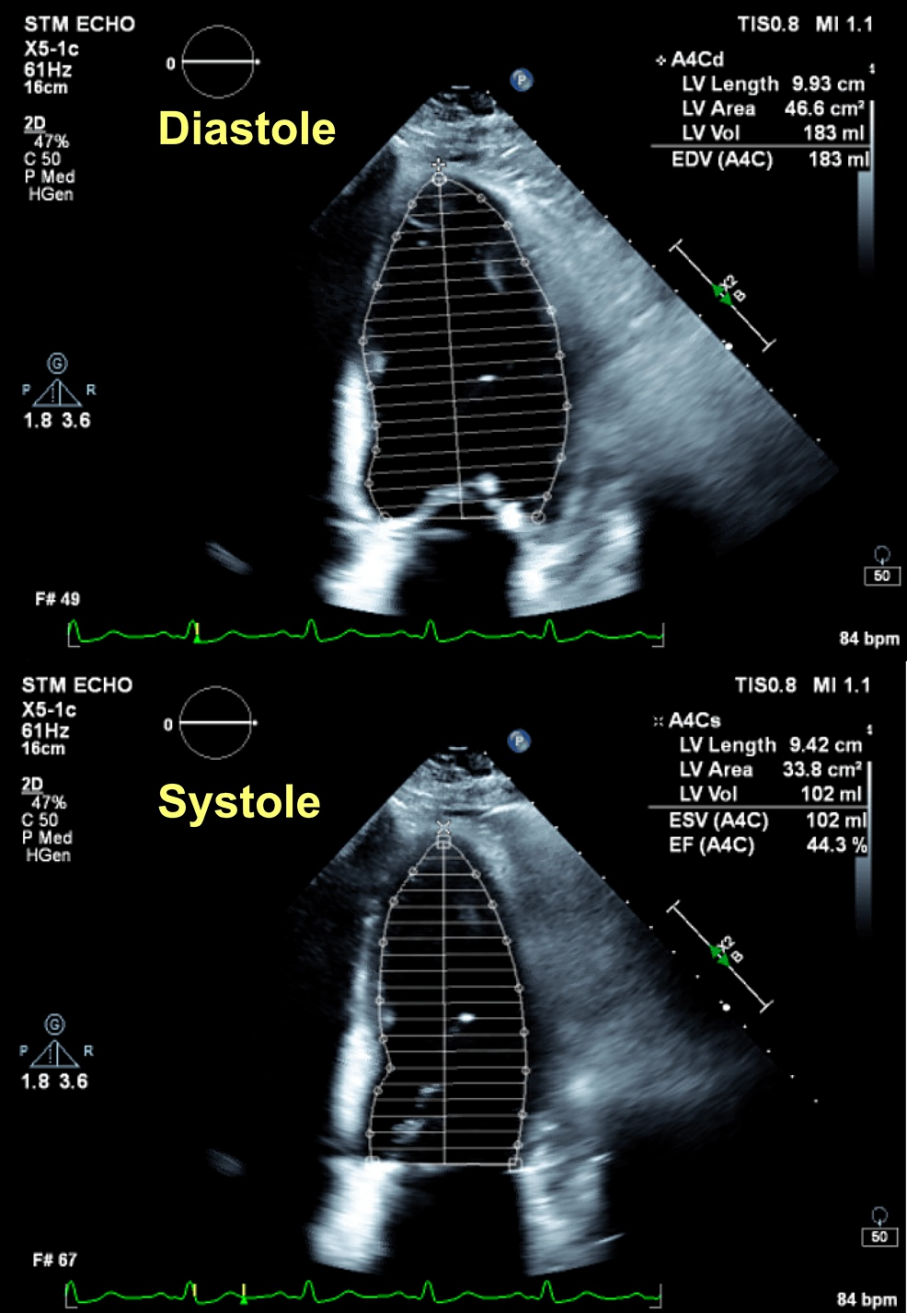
A TTE of the patient during his second admission showing evidence of an improved EF of up to 40%. The EF was measured by a cardiologist and the numbers shown here were inserted by the technician before being reviewed by the cardiologist TTE, transthoracic echocardiogram; EF, ejection fraction
